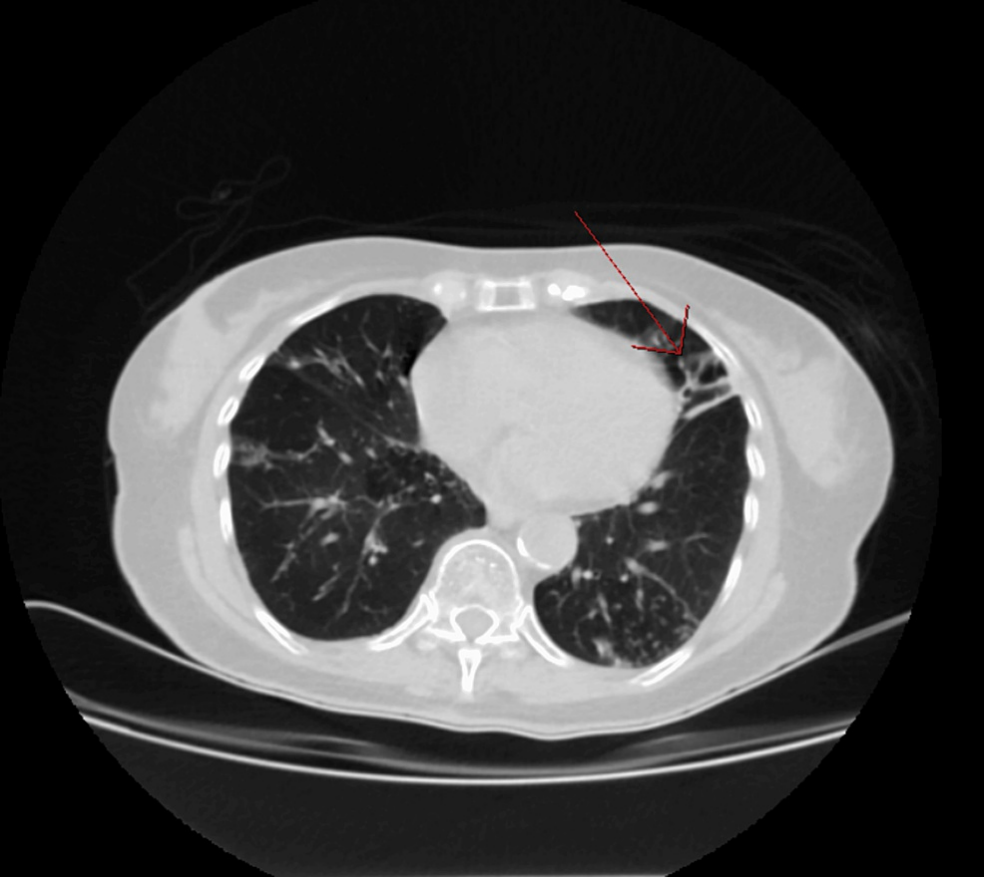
Nodular bronchiectasis involving the lingula of the left lung in CT abdomen/pelvis with intravenous contrast.
